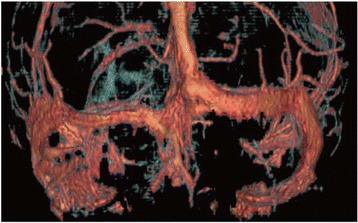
Reconstruction of gadolinium enhanced magnetic resonance imaging performed on admission. Although sinus venous thrombosis was suspected to be the cause of the observed cerebellar infarction with edema, the main venous sinuses were confirmed to be patent
